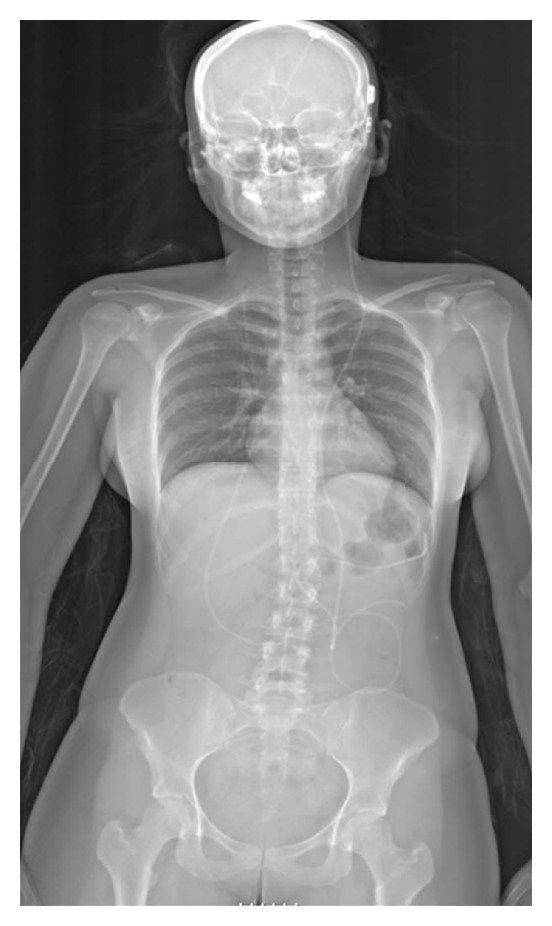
Lodox radiograph showing a ventriculoperitoneal shunt.
